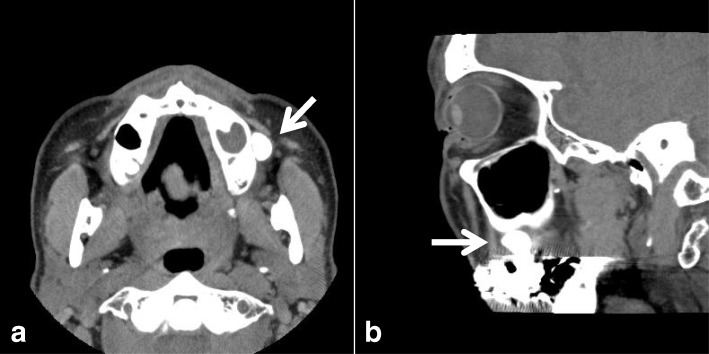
Computed tomography images, a) axial view, b) coronal view, with radiopaque, contrast medium-soaked gauze placed in the cavity which outlines the extent of the pouch (white arrow)
